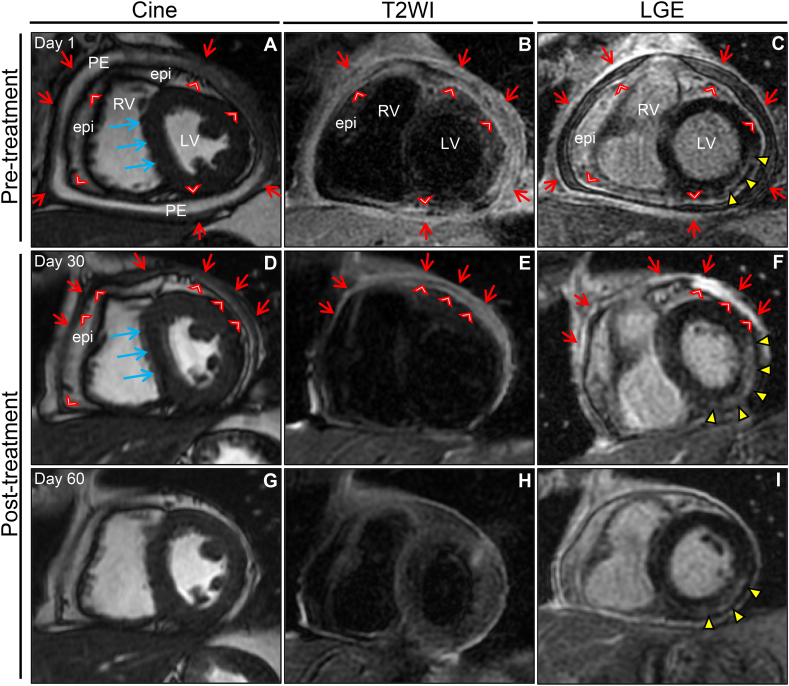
Effects of anti-inflammatory treatment on CMR The cine image shows marked thickening of the entire pericardium and epicardium surrounding the heart (parietal pericardium, red arrow; visceral pericardium, red arrowheads) with a moderate PE and an evident diastolic ventricular septal bounce (blue arrows) on admission (A). Pericardial functional and structural abnormalities persisted despite resolution of the PE after 1 month of treatment (D), which resolved clearly after 2 months of treatment (G). A T2WI image shows diffuse pericardial edema (parietal pericardium, red arrows; visceral pericardium, red arrowheads) observed on admission (B), which persisted after 1 month of treatment (E) and resolved completely after 2 months of treatment (H). LGE image taken on admission (C) shows moderate enhancement of the pericardium (parietal pericardium, red arrows; visceral pericardium, red arrowheads) and partial further intensification (as shown by a bright thick white line) after 1 month of treatment (F) but resolved completely after 2 months of treatment (I). In addition, the LGE in the subepicardial lateral wall of the LV at the papillary muscle level (yellow arrowheads) observed on admission (C) showed further transmural enhancement after 1 month of treatment (F) and remained partially without pericardial LGE after 2 months of treatment (I). CMR, cardiac magnetic resonance; epi, epicardial fat; LGE, late gadolinium enhancement; LV, left ventricle; PE, pericardial effusion; RV, right ventricle; T2WI, T2-weighted image.
